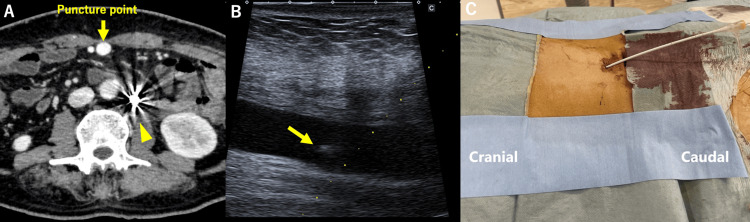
(A) CECT showed a dilated paraumbilical vein (yellow arrow), and MRS was well embolized using coils after the first BRTO (yellow arrowhead). (B) Paraumbilical vein was punctured with a 21G needle under ultrasound guidance. The yellow arrow shows the tip of the needle (yellow arrow). (C) A 7Fr sheath with a tip marker was inserted into the paraumbilical vein in the cranial direction. CECT: Contrast-enhanced computed tomography; BRTO: balloon-occluded retrograde transvenous obliteration; MRS: mesorenal shunt
